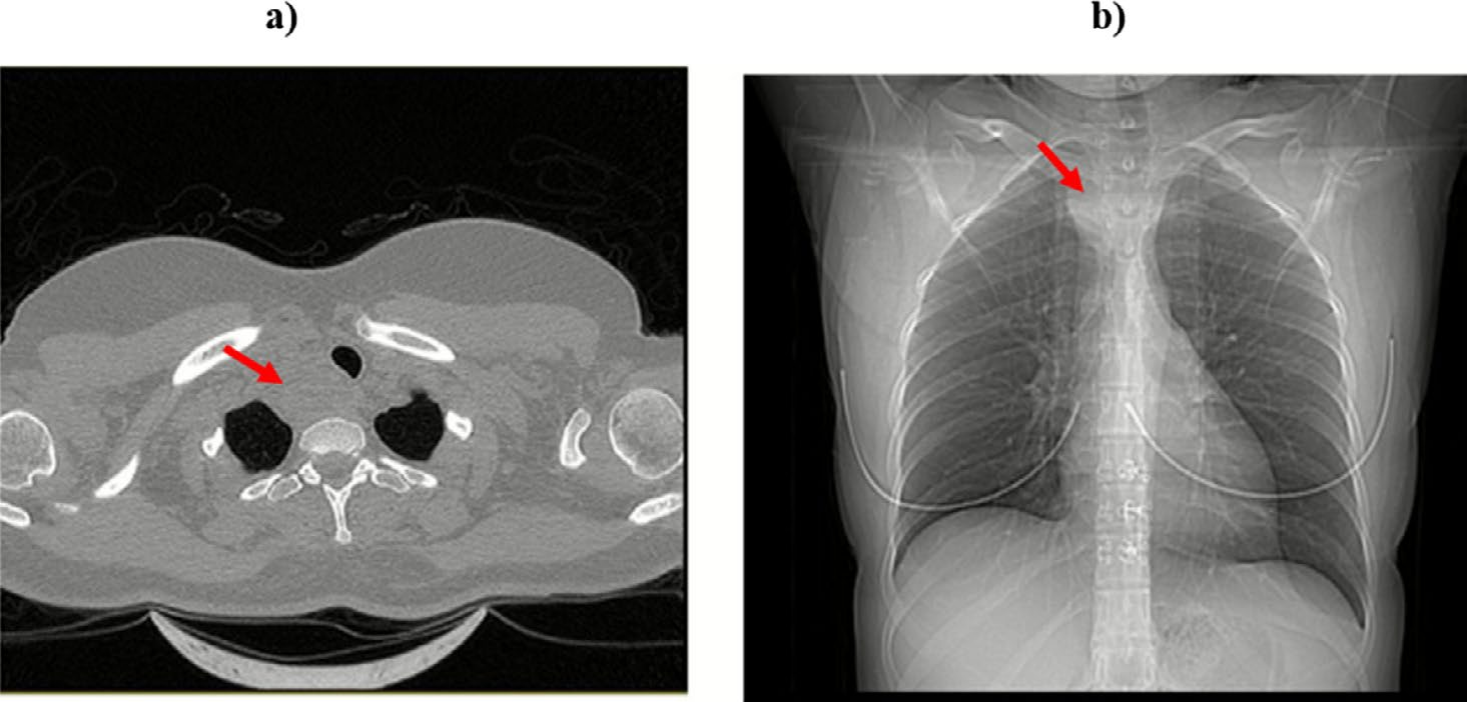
In the superior mediastinal cut, tracheal displacement is noted to the left anterior due to a right parathyroid adenoma. (a) Chest CT scan, and (b) scout view.
